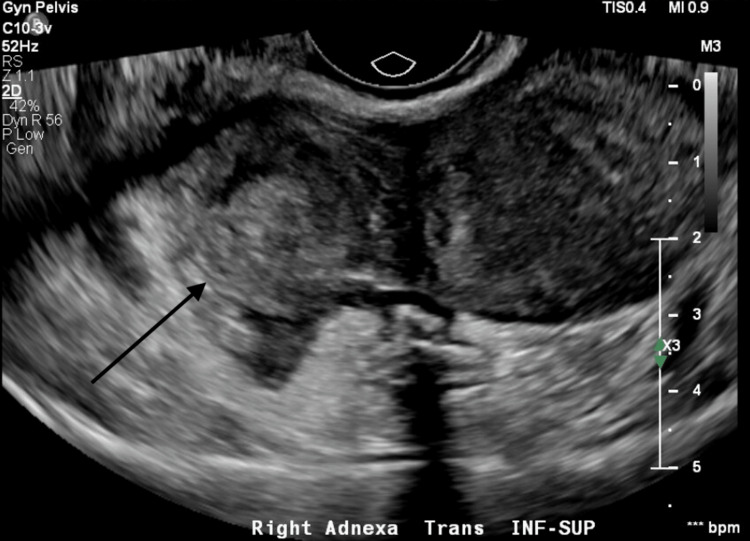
Transvaginal ultrasound of the patient's right adnexa, with heterogenous mass identified (arrow).
